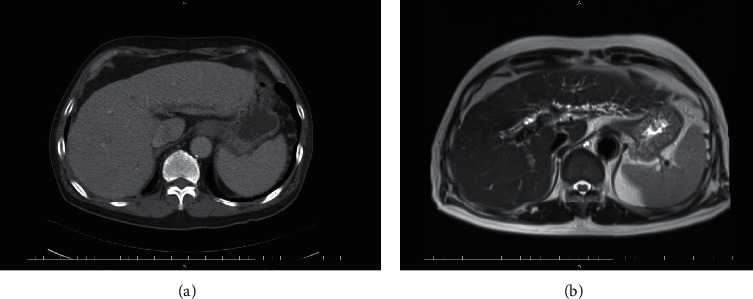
Contrast-enhanced CT (a) and contrast-enhanced MRI (b) did not show any sign of hepatic tumor.
